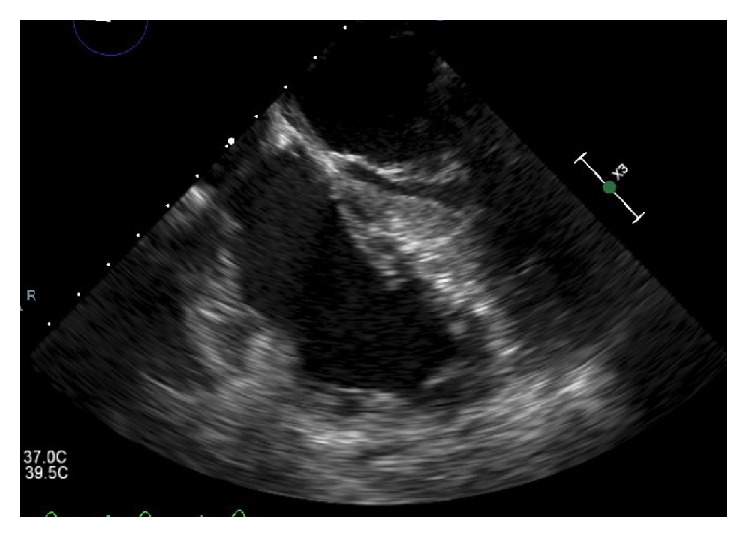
Post-EKOS TEE image (apical four-chamber view) showing resolution of previously noted serpiginous thrombus.
